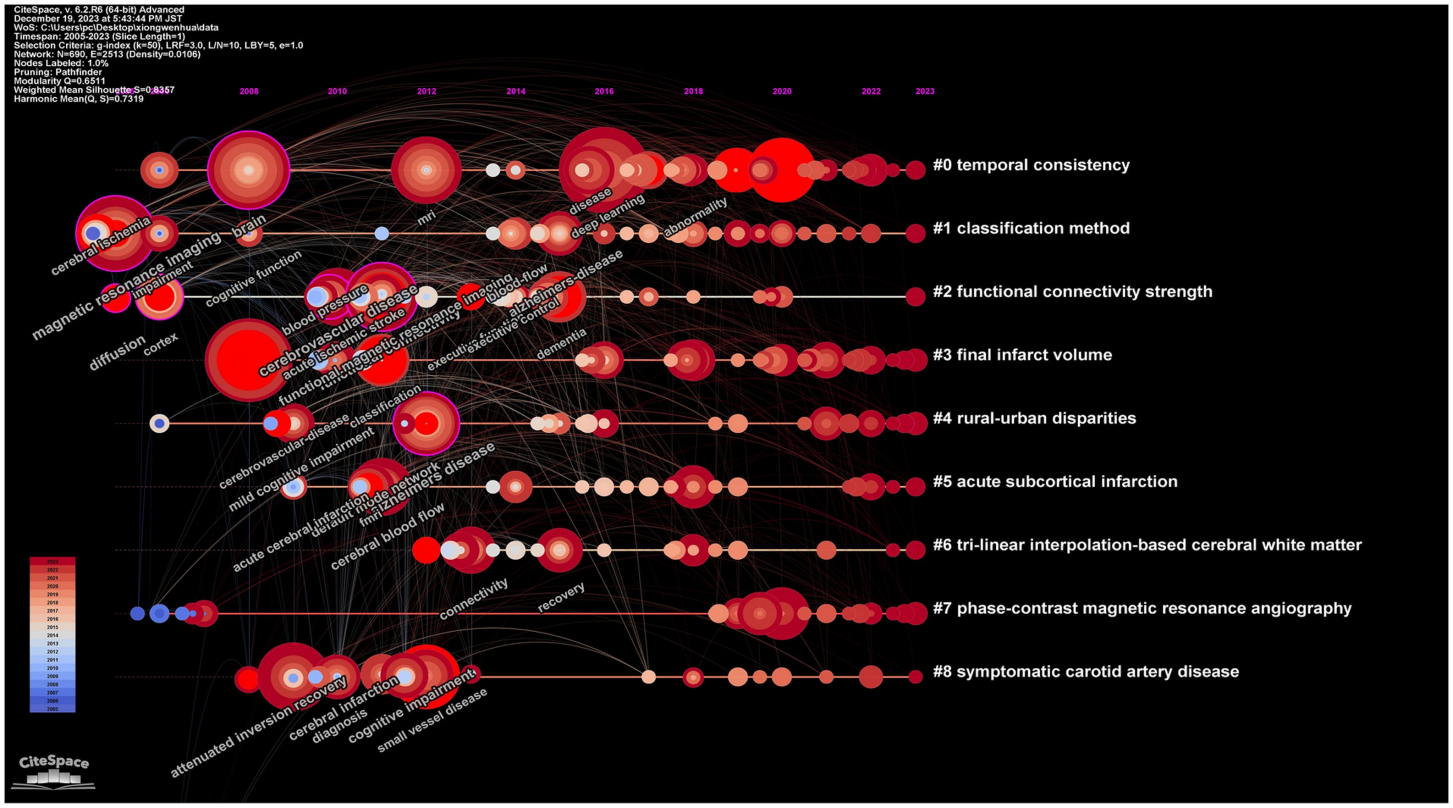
Map of keywords timeline related to machine learning and MRI in early stroke diagnosis.
